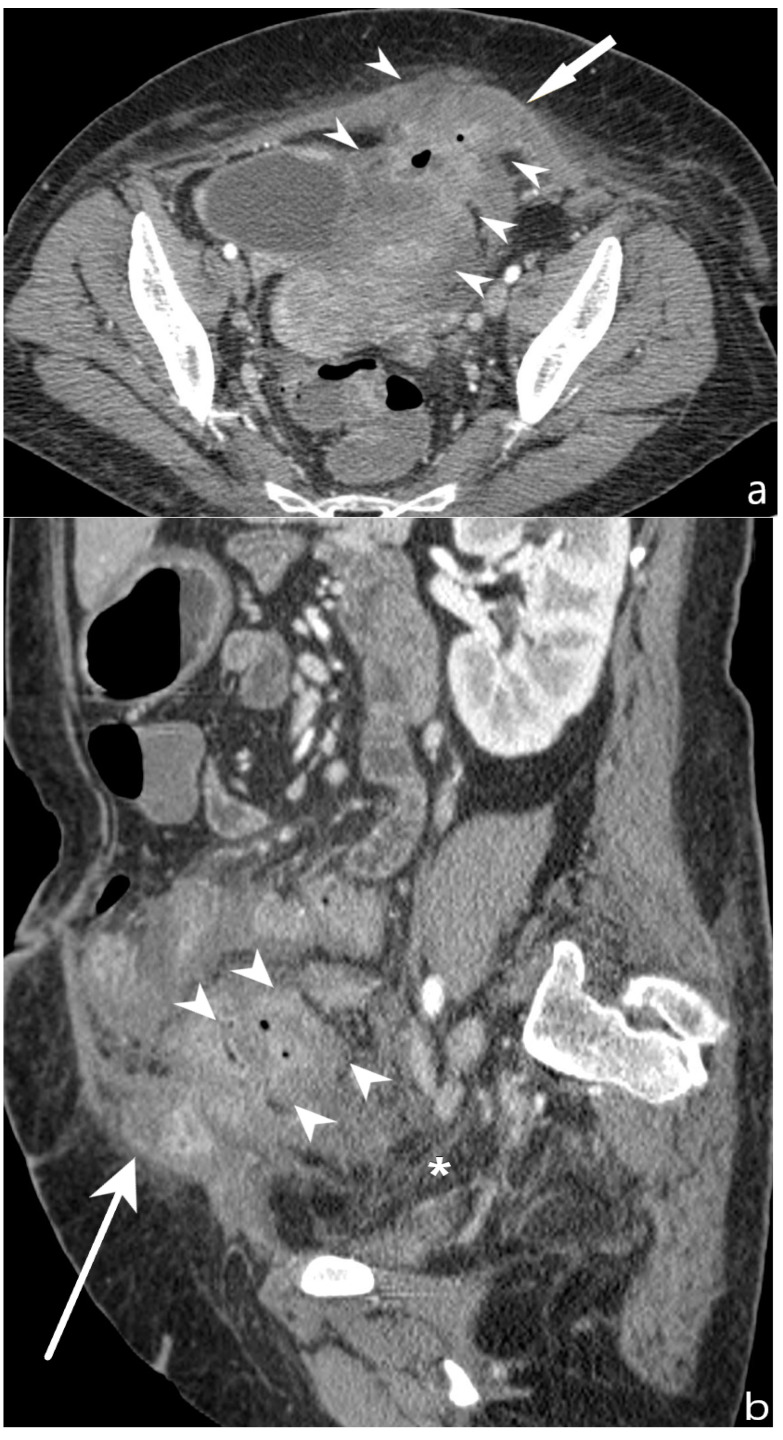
Abscess/phlegmon: CT-E after iodinated contrast injection and administration of polyethylene glycol solution. Axial image (a) shows an abscess/phlegmon (arrowheads) with extension to the wall of the left anterior rectus muscle (white arrow). Sagittal image (b) shows the extension of the abscess/phlegmon (arrowheads) to the wall of the left anterior rectus muscle (white arrow); perienteric fat stranding is present in pelvis (asterisk). Inhomogeneous hypodense tissue portion is present with anti-slope air components. Original figures by LMM and LL.
